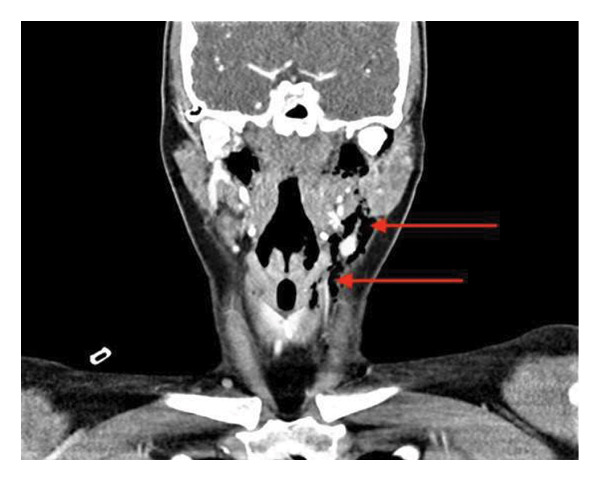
Coronal view of a CT neck, in soft tissue window, showing surgical emphysema (red arrows) situated around the ramus of the left mandible and extending inferomedially to the strap muscles and thyroid cartilage.
